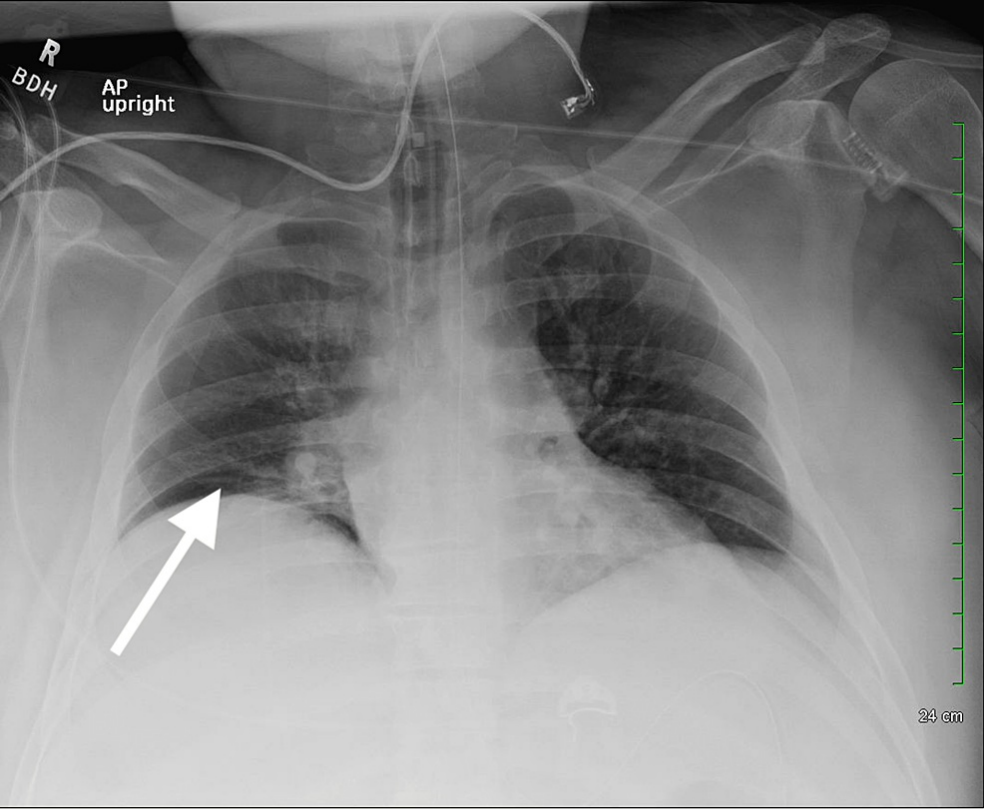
Chest X-ray showing the right basilar sub-segmental atelectasis (arrow)
